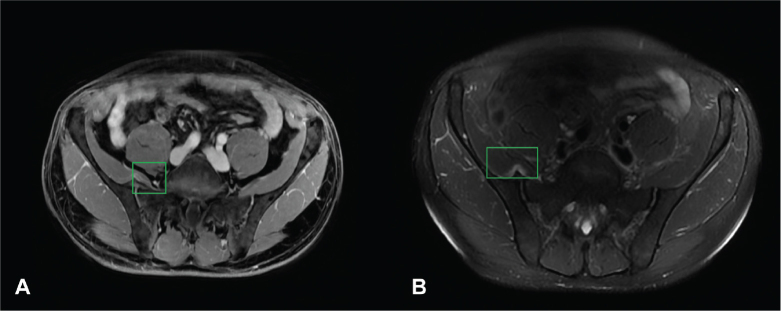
(A) MRI axial Lava-Flex Dixon Water – Gd. (B) MRI axial T2-weighted fat saturated. The imaging demonstrates a synostosis located on the anterior aspect of the sacroiliac joint at the right side, impinging upon the iliopsoas muscle. Associated oedema within the muscle belly is clearly evident as a white area around the synostosis (box: synostosis and oedema).
